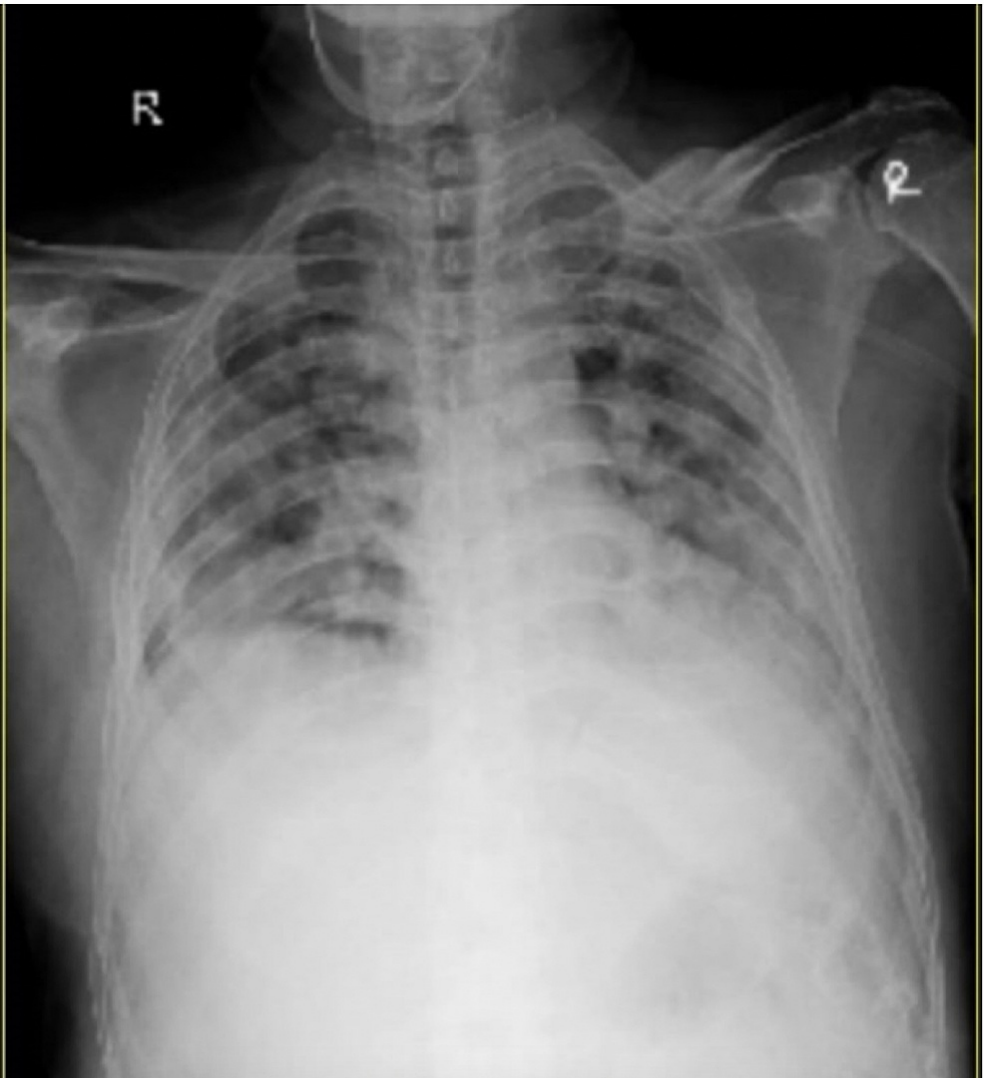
The image is showing the appearance of new infiltrates on the chest x-ray after the chest pain episode
